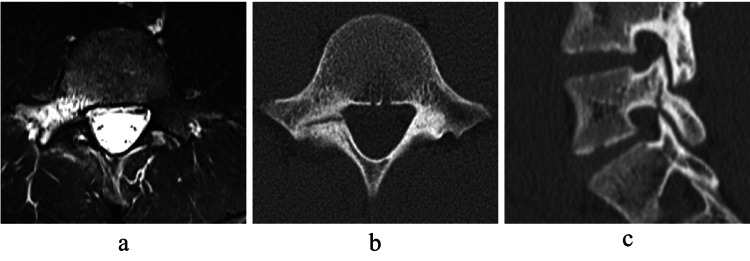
Imaging at 6 months after first examination Six months after the first examination, magnetic resonance images (MRI) showed high signal change (a), and computed tomography (CT) showed a hairline in axial slice (b) and an incomplete fracture in sagittal slice (c).
